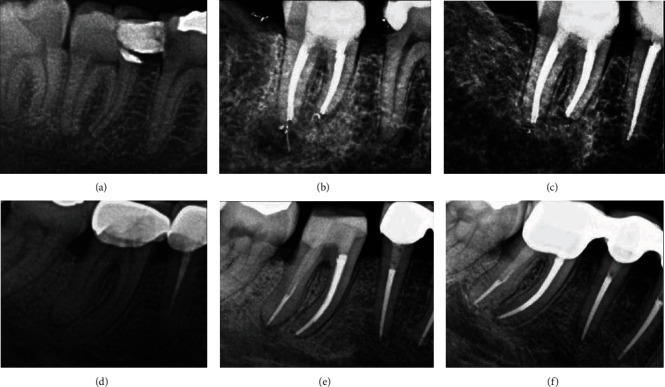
Radiographic evaluation of different teeth before and after the treatment: (a) right mandibular second molar tooth, preoperative radiograph; (b) right mandibular second molar tooth, after the treatment; (c) right mandibular second molar tooth, a 68-month follow-up; (d) right mandibular first molar tooth, preoperative radiograph; (e) right mandibular first molar tooth, after the treatment; (f) right mandibular first molar tooth, a 50-month follow-up.
